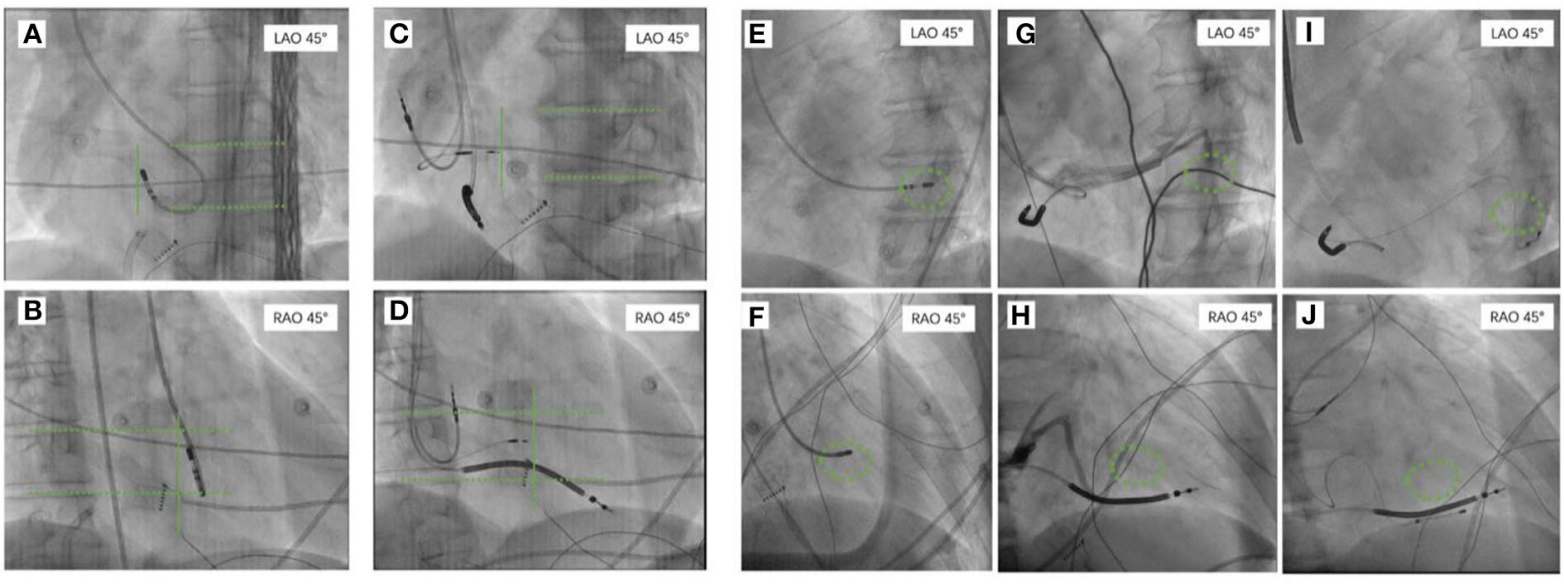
The tip of the mapping catheter under X-ray recorded at the right anterior oblique (RAO) 30° position and left anterior oblique (LAO) 45°. For patients who were planning to undergo LBBAP, the tip of the mapping catheter at the area of the left bundle branch in the left ventricle was recorded at RAO 30° and LAO 45° as references (A,B). The 3,830 electrode was implanted on the right ventricular septum surface corresponding to the tip of the ablation catheter at RAO 30° and LAO 45° (C,D). For patients who were planning to undergo CVP, the tip of the mapping catheter that was placed on the intima surface of the last activation of the left ventricle was recorded at RAO 30° and LAO 45° as references (E,F). Coronary venography was performed to identify the cardiac vein (G,H) closest to the tip of the ablation catheter as the target for implantation of the left ventricular electrode (I,J). RAO, the right anterior oblique; LAO, the left anterior oblique; CVP, coronary venous pacing; LBBAP, left bundle branch area pacing.
